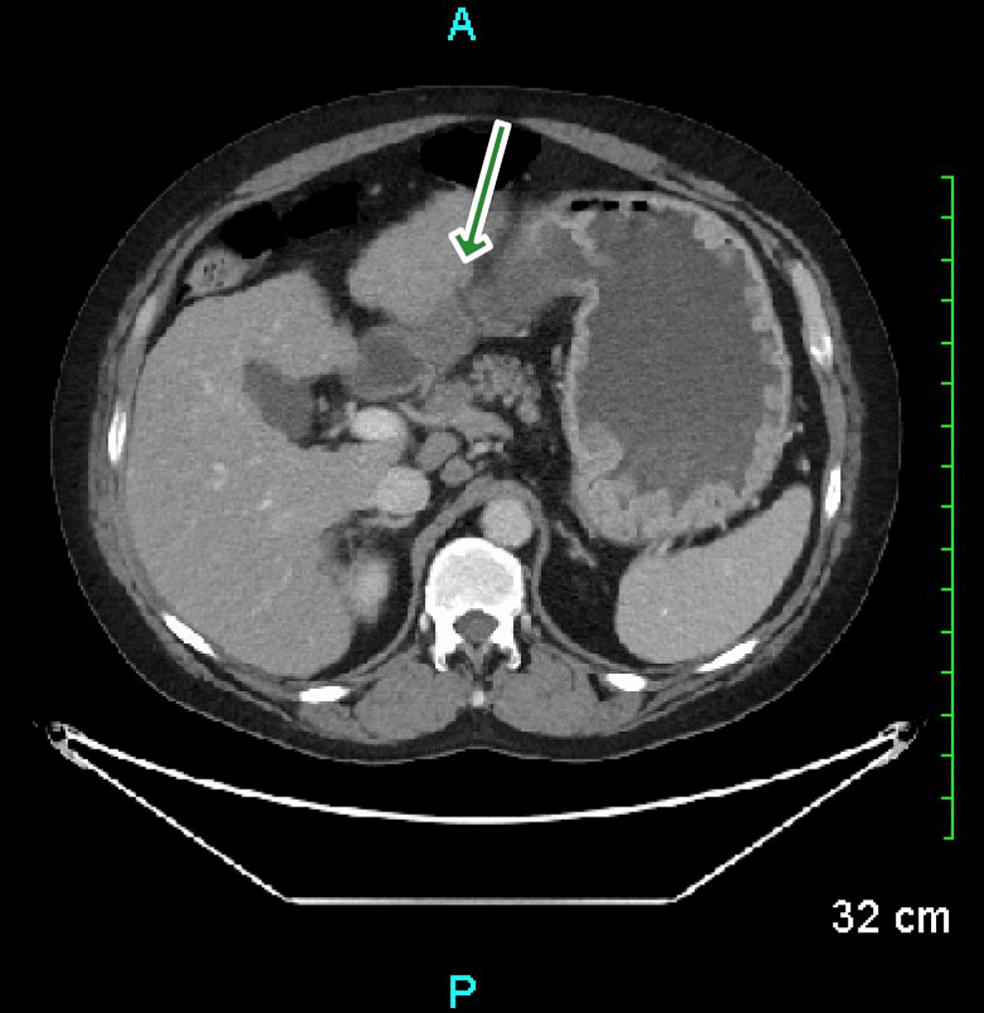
CT Abdomen The green arrow pointing towards an apple-core lesion in the duodenum.
